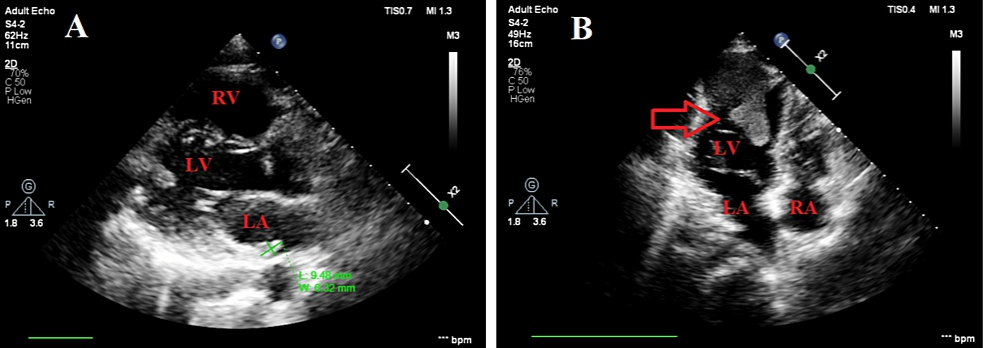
Transthoracic echocardiographic images of the left atrial and ventricular masses A: View of the left atrial mass in the parasternal long-axis window. B: Image of the left ventricular mass in the apical four-chamber window. LA: left atrium; LV: left ventricle; RV: right ventricle; red arrow: LV apicolateral mass.
